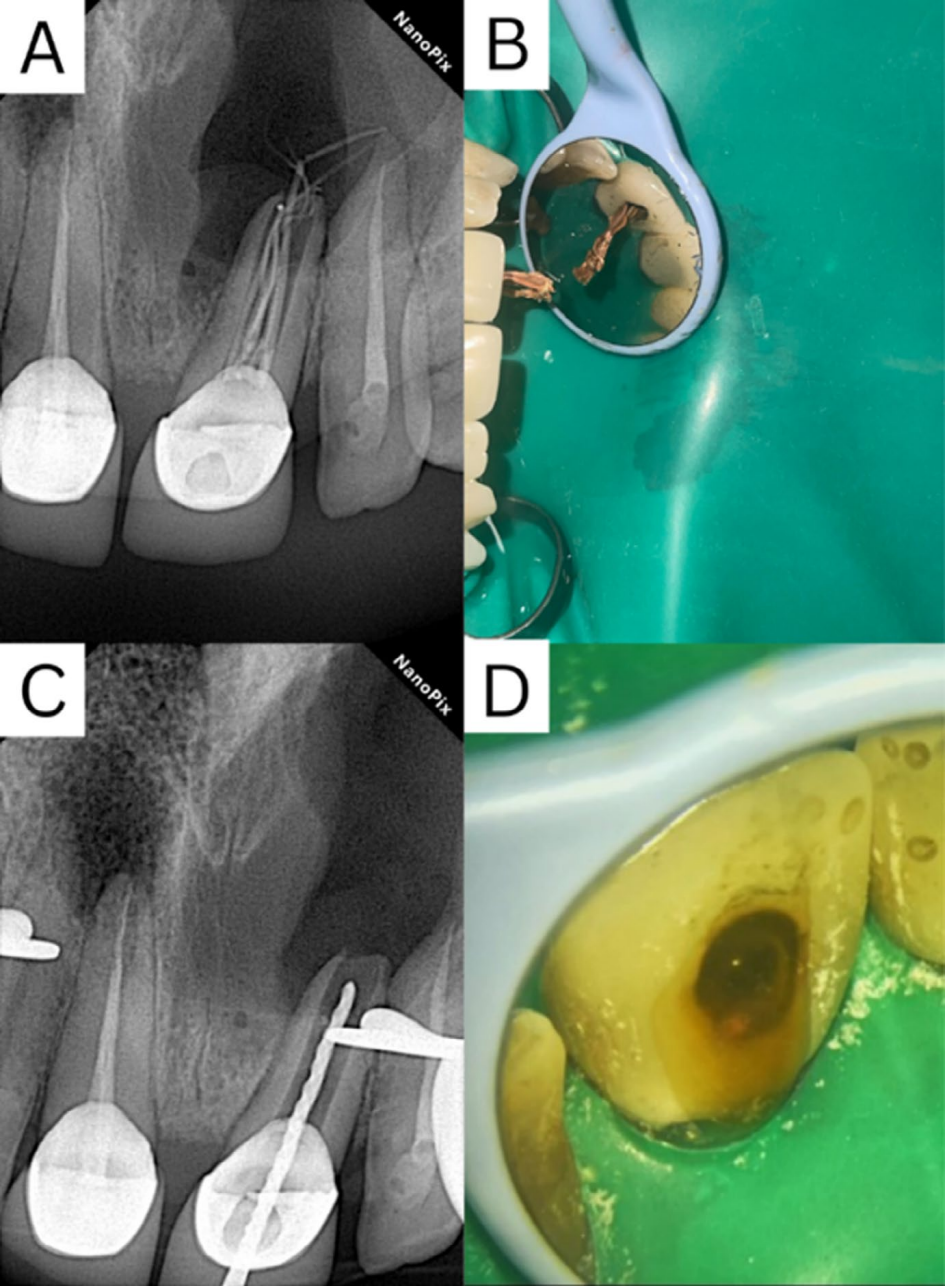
(A) Initial periapical radiograph showing insufficient obturation and the presence of a lesion at the apex of the upper right central incisor. (B) Removed gutta‐percha (C) Periapical radiograph showing the complete removal of the previous fillings. (D) Clinical picture showing persistent pus flow from the access site, indicating ongoing infection.
